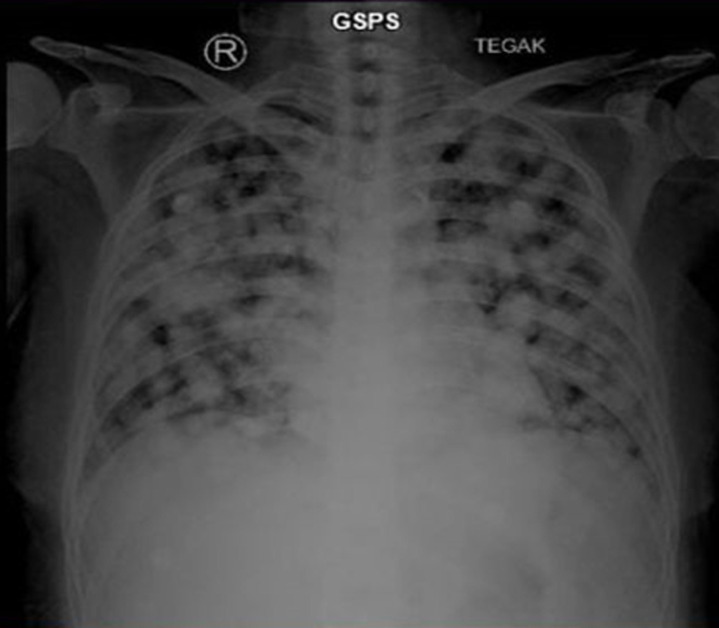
chest X-ray revealing metastasis to the lungs
